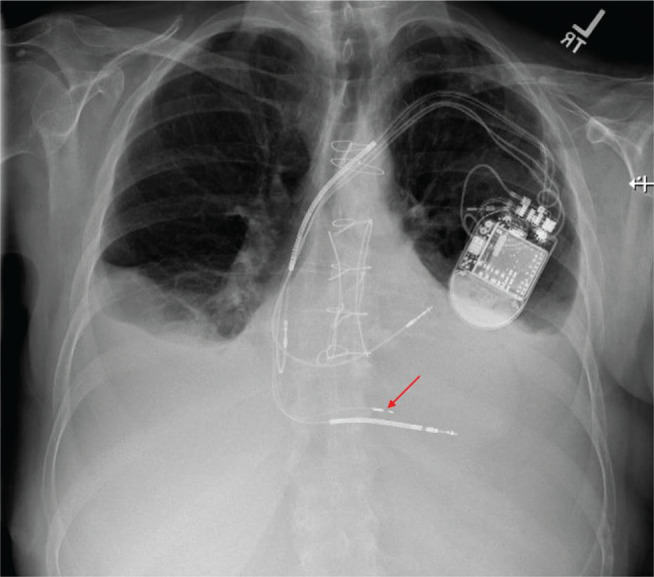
Chest X-ray anteroposterior view demonstrated the location of the left bundle branch pacing lead (arrow).
